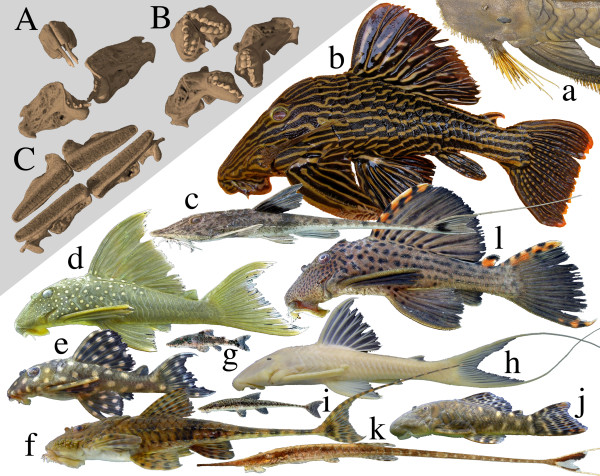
Representative morphological diversity within Loricariidae. Inset: CT reconstructions of upper and lower jaws of (A) *Leporacanthicus* (an insectivore); (B) *Panaque* (a wood-eater); and (C) *Chaetostoma* (a detritivore-algivore). Photos (scaled to approximate relative size): (a) *Pseudancistrus pectegenitor* (Ancistrini), (b) *Panaque armbrusteri* (Ancistrini), (c) *Pseudohemiodon* sp. (Loricariini), (d) *Hemiancistrus subviridis* (Ancistrini), (e) *Hypancistrus contradens* (Ancistrini), (f) *Spatuloricaria* sp. (Loricariini), (g) *Parotocinclus eppleyi* (Hypoptopomatinae), (h) *Hemiancistrus pankimpuju* (Ancistrini), (i) *Oxyropsis acutirostra* (Hypoptopomatinae), (j) *Chaetostoma* sp. (Ancistrini), (k) *Farlowella* sp. (Farlowellini), (l) *Leporacanthicus triactis* (Ancistrini). Fish photos by N. K. Lujan (*b*,*g*,*h*,*i*,*j*,*k*) and M. H. Sabaj Pérez (*a*,*c*,*d*,*e*,*f*,*l*).
